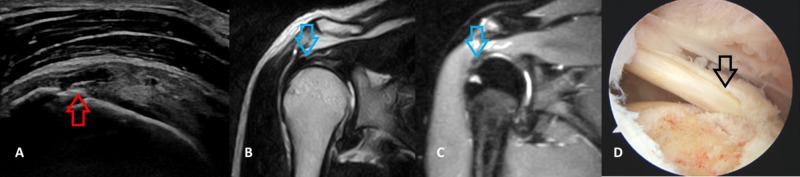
A 36-year-old male patient with a bursal partial-thickness supraspinatus tear A: longitudinal HRUSG image of the supraspinatus tendon reveals a hypoechoic discontinuity of the supraspinatus tendon extending on the bursal surface and extending up to the greater tuberosity and not to the articular surface (red arrow). Note the cortical irregularity. B and C: T2 & STIR coronal MRI image of the shoulder joint reveals a partial-thickness tear in the supraspinatus muscle (blue arrow). The arthroscopic image demonstrates atotalbursal partial-thickness supraspinatus tear (black arrow). HRUSG - high-resolution ultrasonography; STIR - short TI Inversion Recovery
